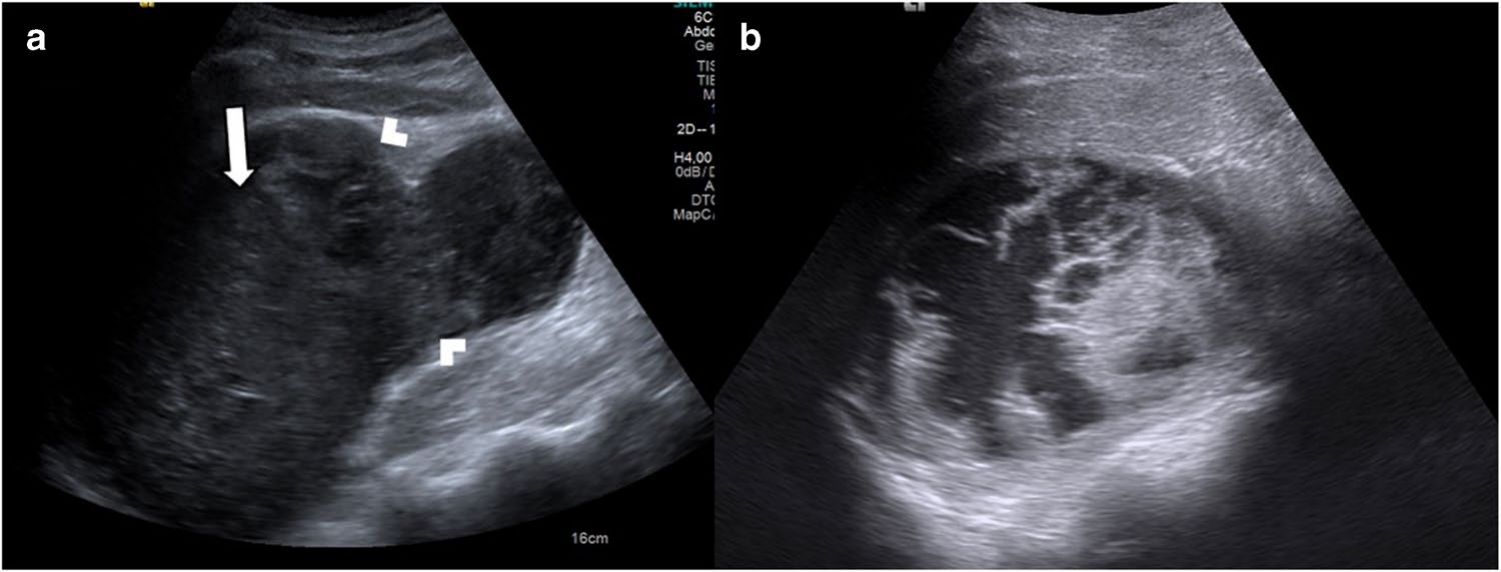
Spontaneous subcapsular hematoma. A 15-year-old boy presented to the Emergency Department with acute left flank pain. a Axial grayscale ultrasound (US) image reveals a mildly heterogeneous solid mass (*arrow*) compressing the left renal parenchyma (*arrowheads*). Color Doppler US revealed no vascularity within the lesion (not shown). b Follow-up axial grayscale US image performed 2 months later demonstrates a decrease in the lesion size with partial liquefaction. The underlying etiology remained unclear
